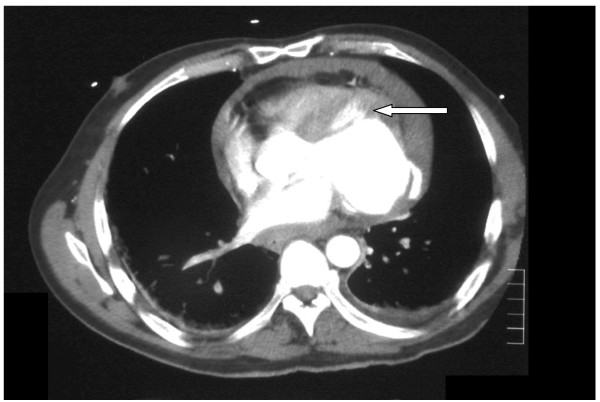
CT scan showing leakage of contrast from the left ventricle free wall rupture (arrow).
